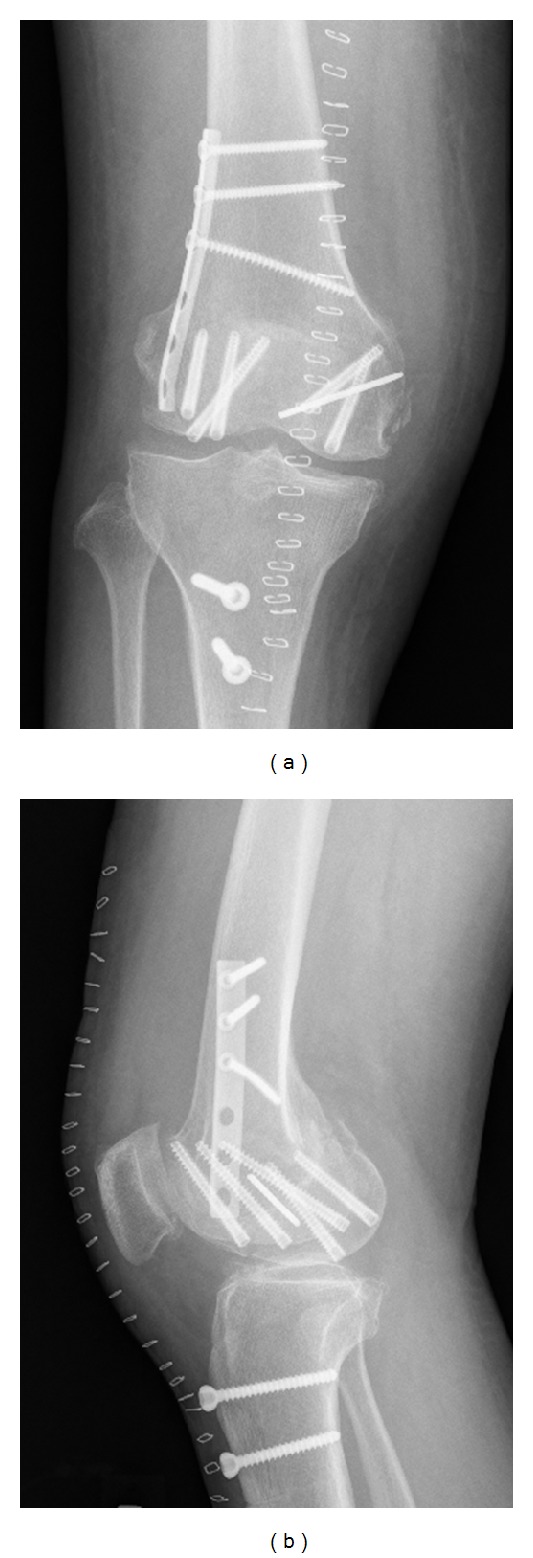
Anteroposterior (a) and lateral (b) radiographs obtained right after surgery.
